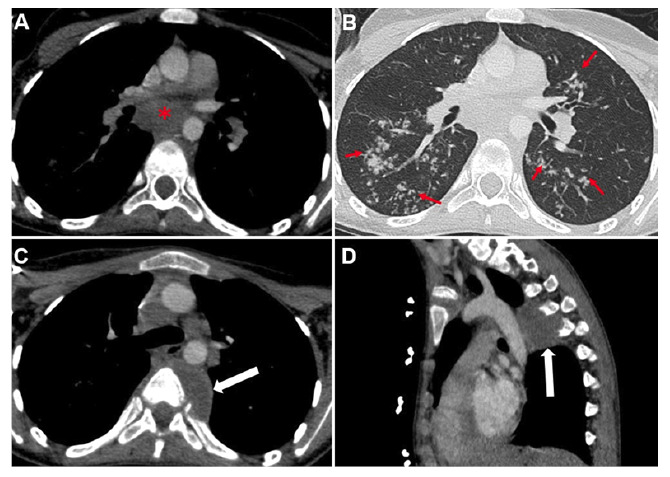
Axial non-contrast chest CT, soft tissue window (A), demonstrates necrotic lymphadenopathy (asterisks) in the mediastinum and bud-like branch patterns, as well as patchy consolidated areas (arrows) in the bilateral parenchyma on the parenchymal window (B). Axial (C) and sagittal (D) non-contrast chest CT images show a hypodense lesion causing rib destruction in the left paravertebral region (arrow).
